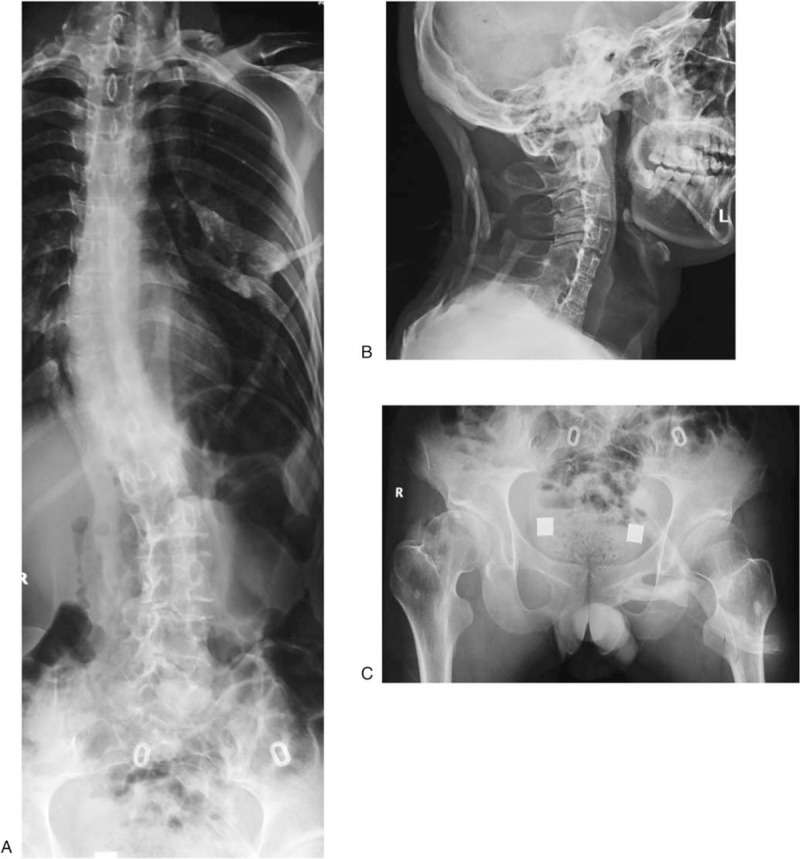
(A) X-ray and magnetic resonance imaging showed fusion anomalies of heterotrophic ossification at the posterior elements of the cervical and upper thoracic spine, scapula, lumbar paraspinal muscle area, and hip area. (B) Lateral cervical spine radiography demonstrated osseous bridging of the posterior elements. (C) Anteroposterior hip X-ray showed a short, broad femoral neck on both sides with bridge-like heterotopic ossifications extending across the left hip joints and iliac bone as well as lateral to the thigh.
